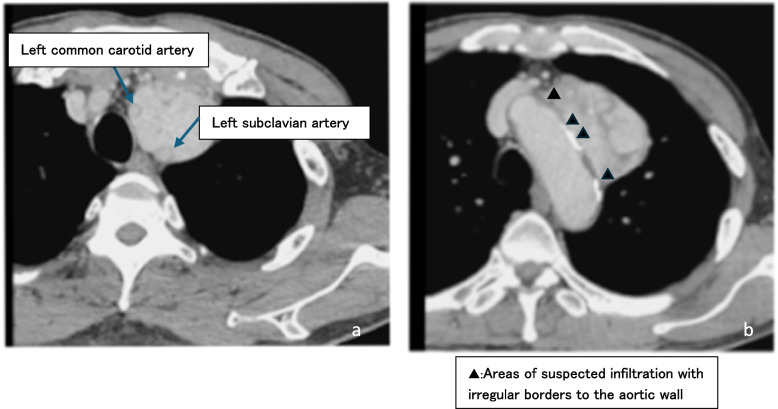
Enhanced chest computed tomography scan
